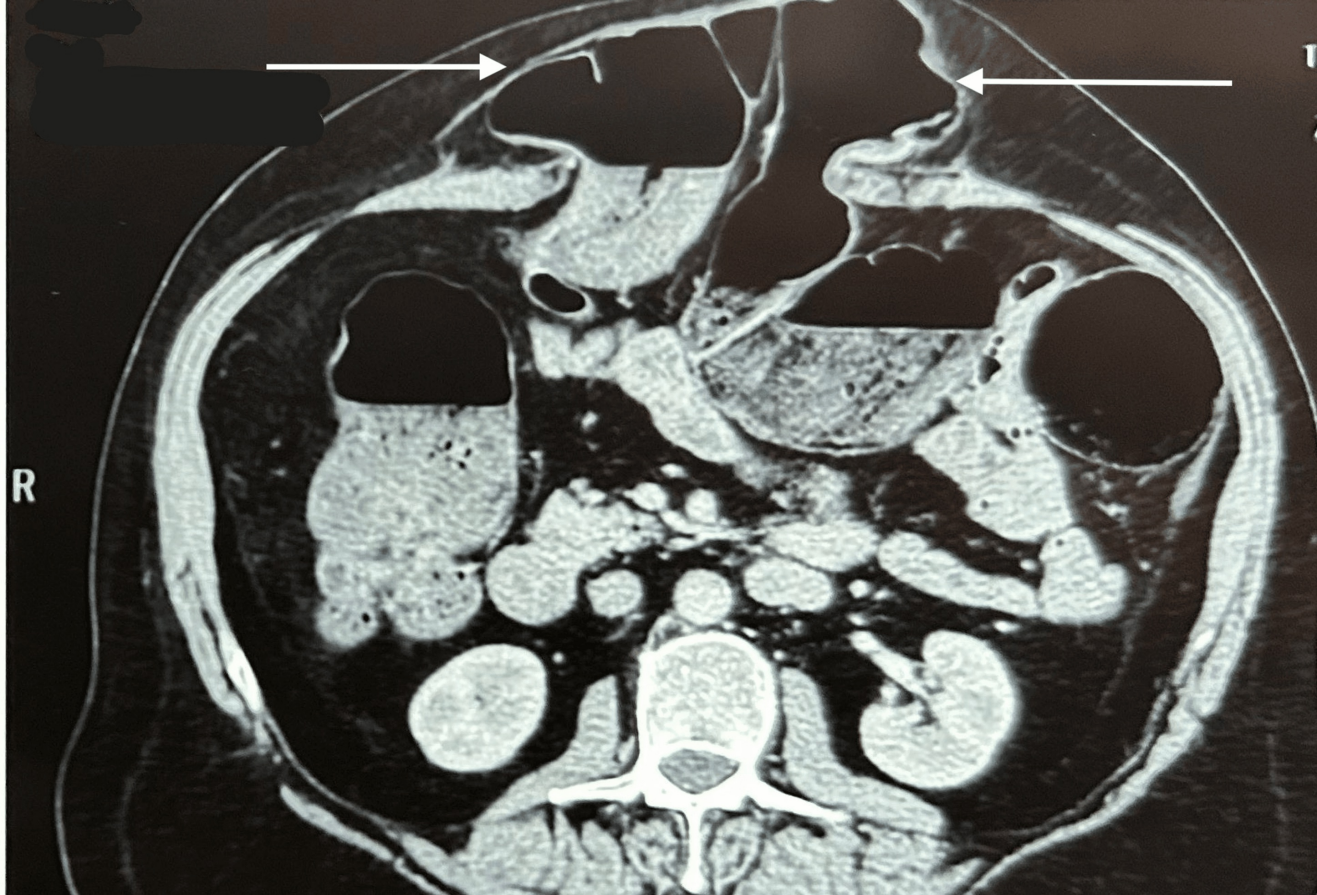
CECT showing previous incisional hernia with herniating large bowel, small bowel, and omentum in a large midline defect: M2-3, W3 CECT: contrast-enhanced computed tomography
